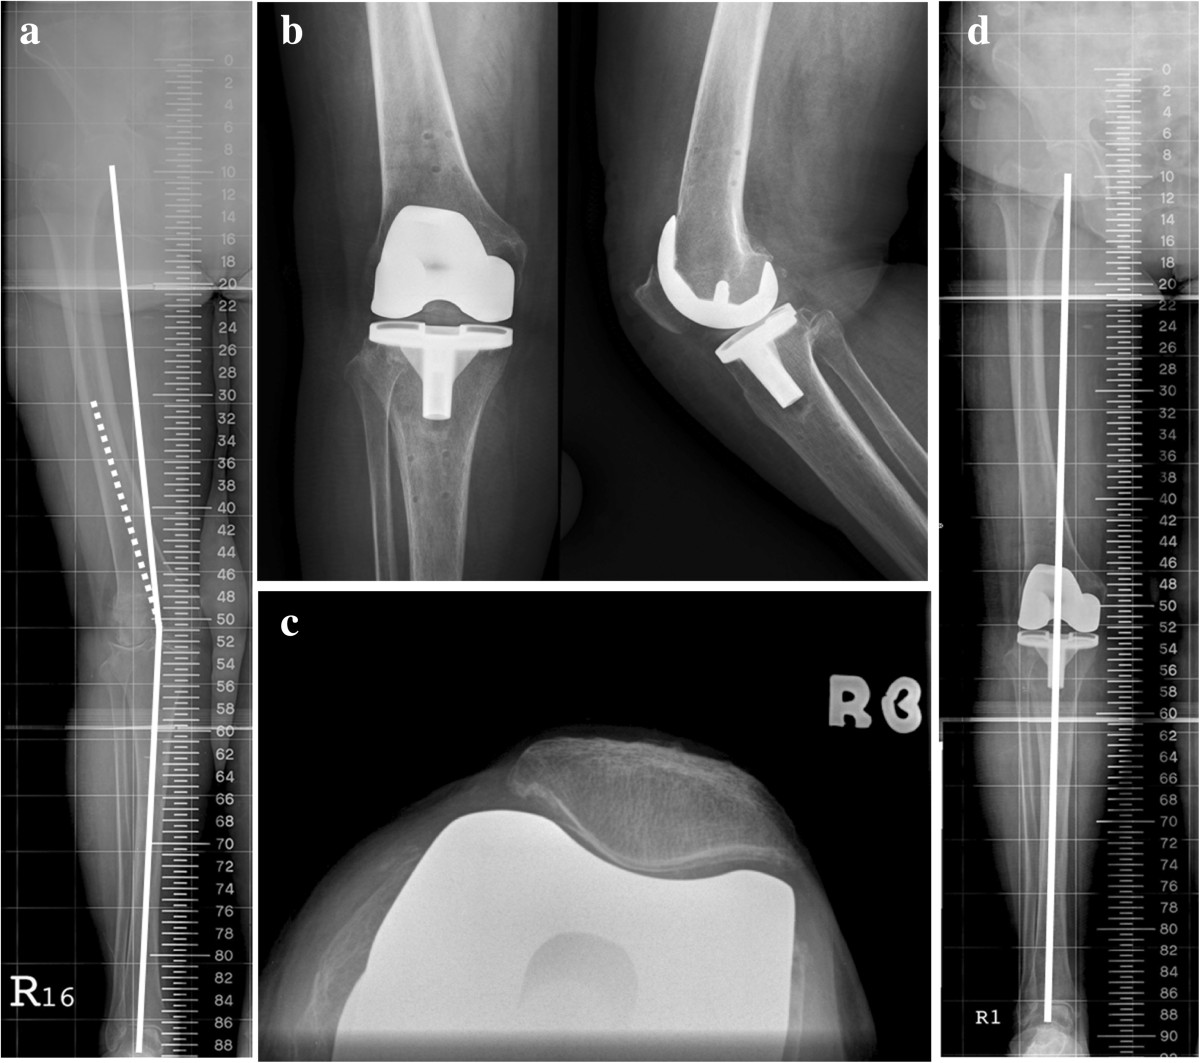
A 74-year female with advanced valgus deformity of the right knee joint. a. The preoperative hip-to-ankle standing radiography showing significant genu valgus deformity with preoperative anatomical axis of 16.1°. b. Radiograph after total knee replacement with a cruciate-retaining type prosthesis. c. Radiograph of post-operative patella axial view after total knee replacement with a cruciate-retaining type prosthesis. d. Postoperative radiograph showing complete restoration of limb alignment after undergoing CN-TKA.
